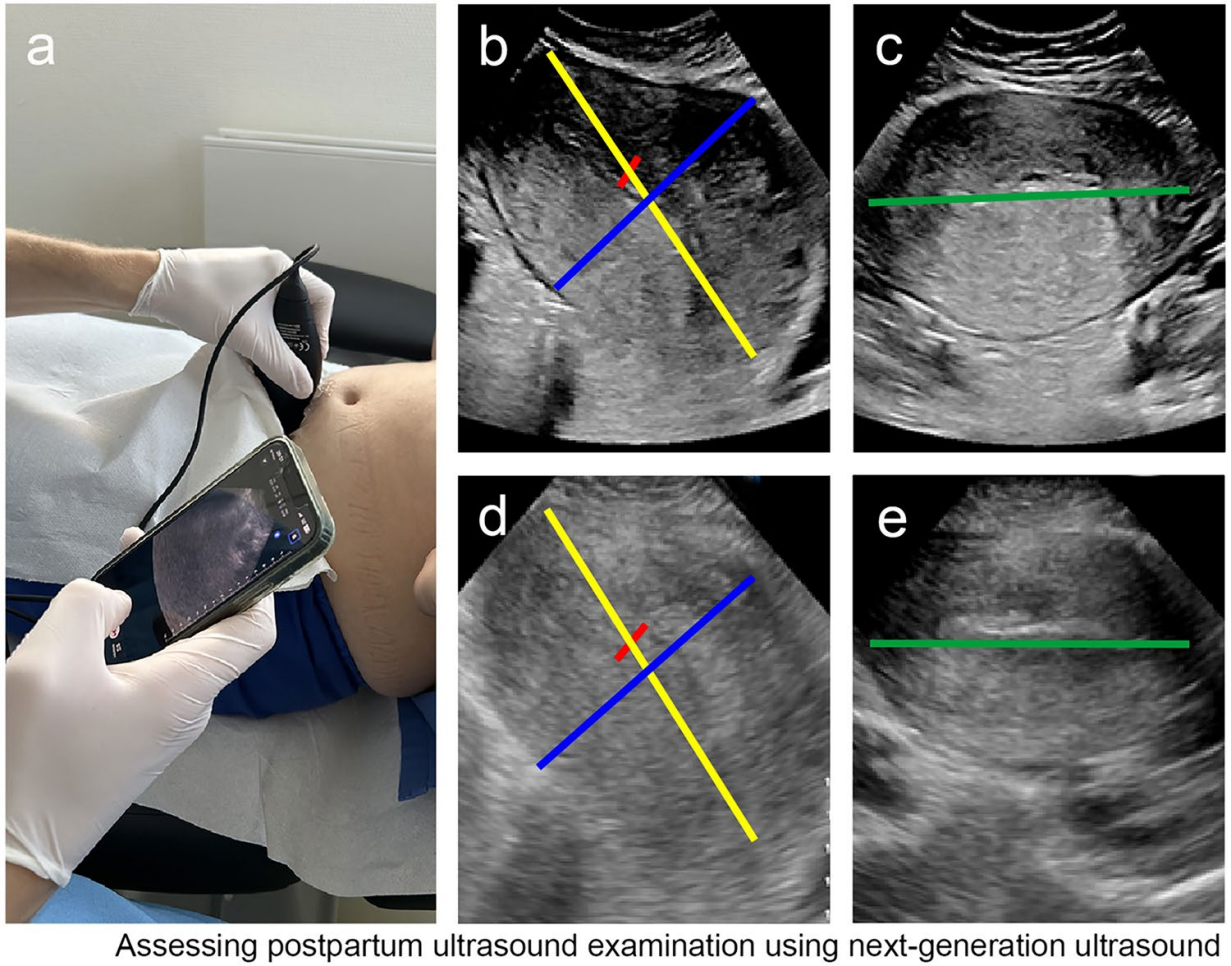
Images of an examination with a portal ultrasound machine (a) of transabdominal ultrasound scans using a standard ultrasound machine based on piezo technology (b–c), and of a portable ultrasound machine based on silicon chips (d–e): demonstration of the sagittal scan plan (b, d) showing the sagittal diameter (SA, yellow), the longitudinal diameter (LU, blue), the diameter of the cavum (LC, red), and of the transversal scan plan (c, e) showing the transversal diameter (HU, green)
